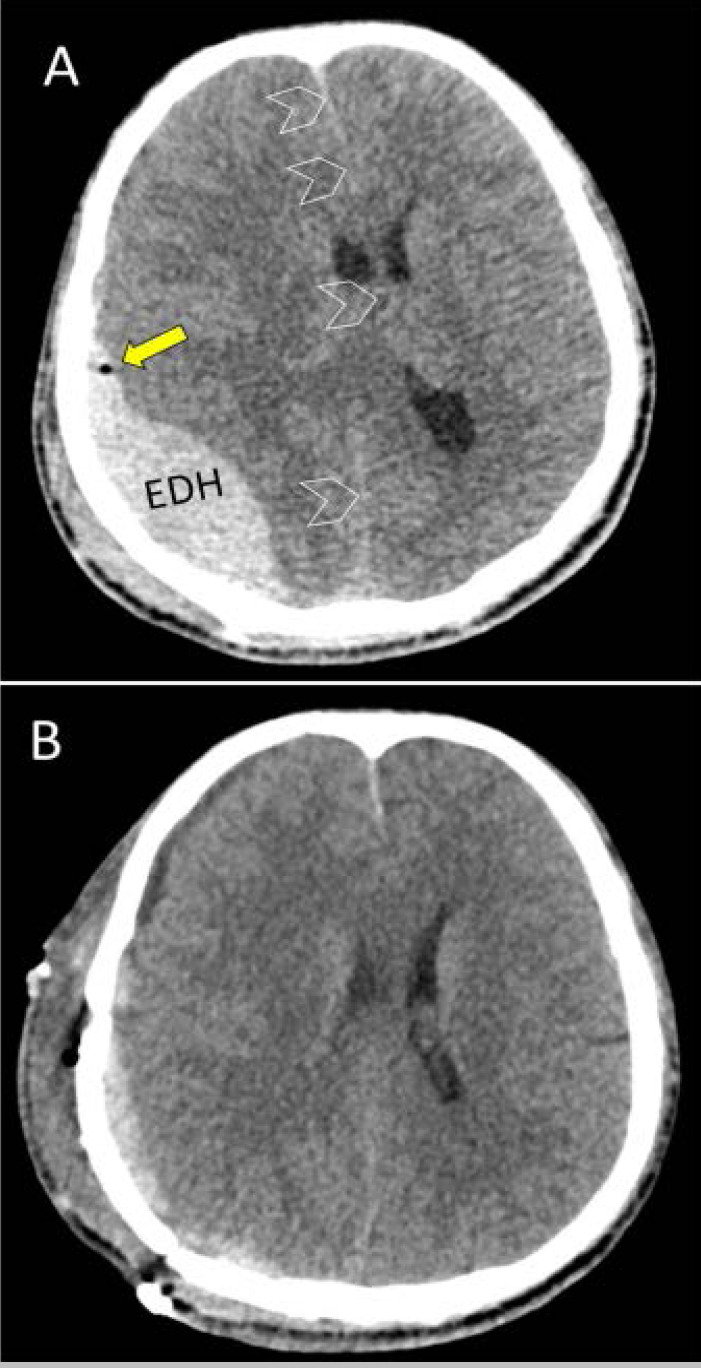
A 29-year-old camel caregiver fell off a camel, followed by being stepped on his head by the camel's hoof. Axial CT scan (A) of the head demonstrates right-sided parieto-occipital epidural hematoma (EDH) with midline shift (arrowheads) associated with intracranial air (yellow arrow) and hematoma of the right scalp. Postoperative day one CT scan (B) following right craniotomy showing a significant reduction of EDH.
